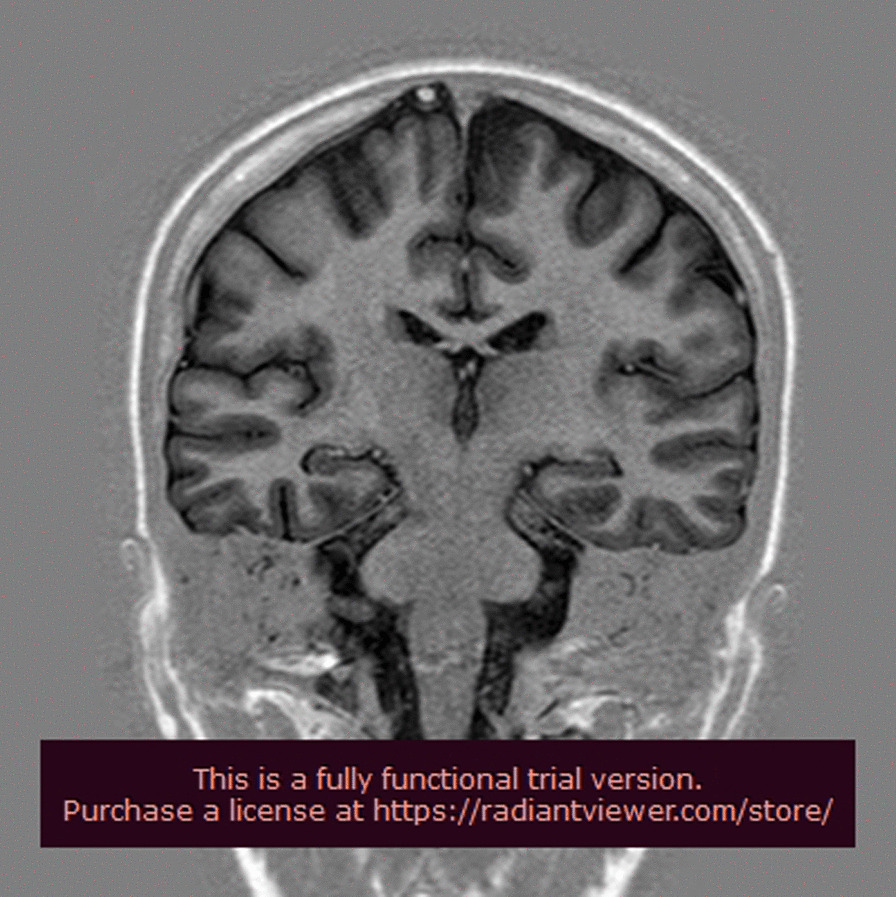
Brain magnetic resonance imaging showing normal findings
